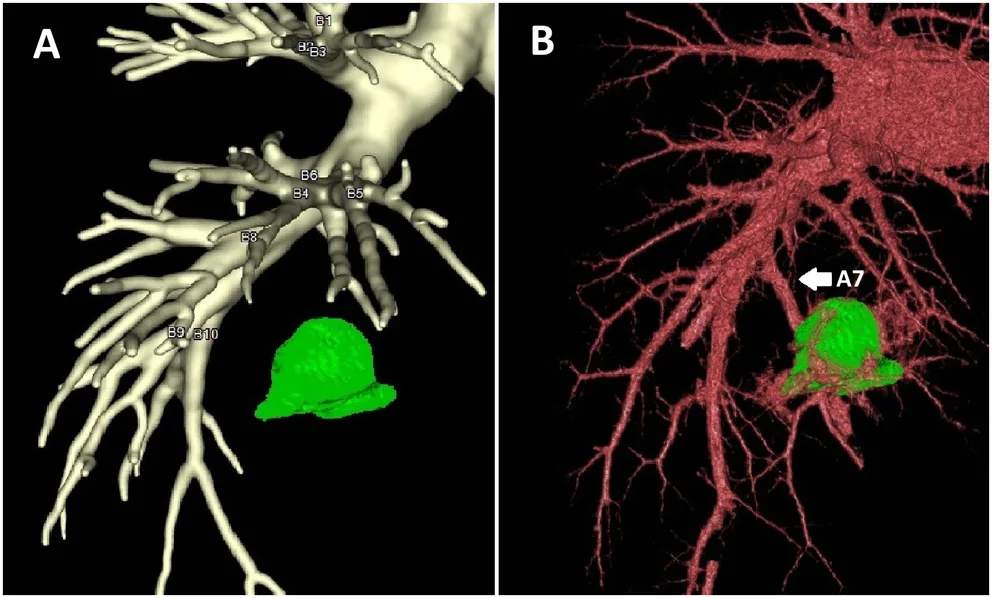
Three‐dimensional computed tomography reconstruction of the bronchial and pulmonary arterial anatomy. (A) Bronchial reconstruction demonstrating interruption of the right B7 bronchus. (B) Pulmonary arterial reconstruction showing a normally branching segmental artery (A7) (arrow) supplying the lesion.
